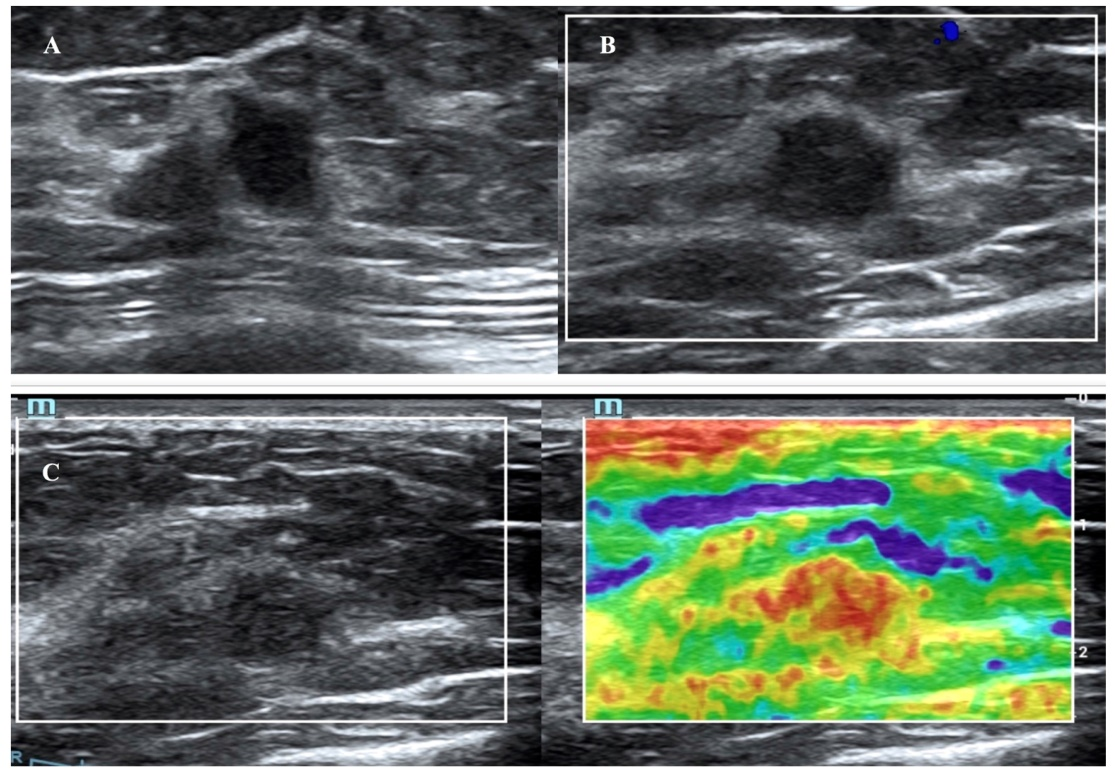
A breast mass from a 64-year-old woman with invasive carcinoma, which was classified as US BI-RADS category 4b and MRI BI-RADS category 3. B-mode US showed a 1.3-cm hypoechoic mass (A). The color Doppler ultrasound image of the same mass reveals no internal or peripheral blood flow signal (B). The elastographic image showed that the lesion was scored as a 5 with the 5-point method (C).
